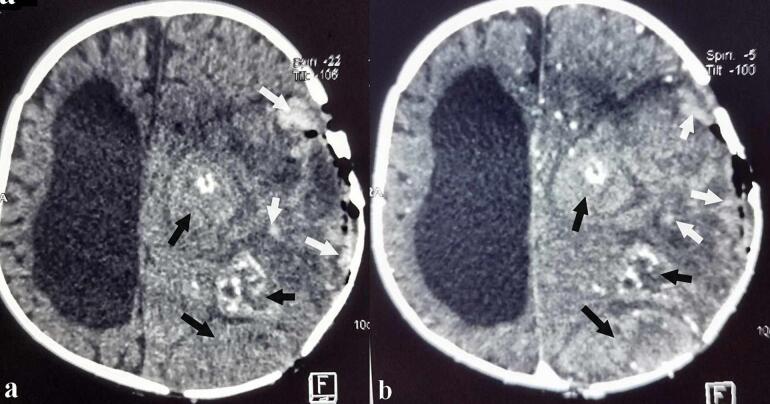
Axial CT scan in parenchymal window performed 24 h postoperatively before (a) and after enhancement (b) showing the tumor residue (black arrows) with some hemorrhagic foci (white arrows). Note the presence of fine calcifications not clearly visible on preoperative MRI scans and the calvarial defect opposite the pneumocepalus.
